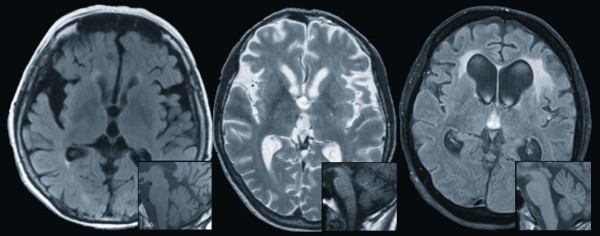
Magnetic resonance images. MRI show an asymmetric frontotemporal atrophy on transversal scans in case 1 (left, FLAIR sequence), case 2 (middle, T_2 _sequence), and case 3 (right, FLAIR sequence). In inboxes a relatively preserved brain stem is demonstrated on sagittal sections.
